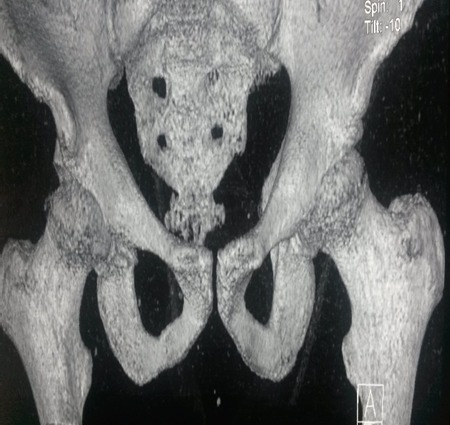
Post reduction CT showing no fracture of the femoral head
